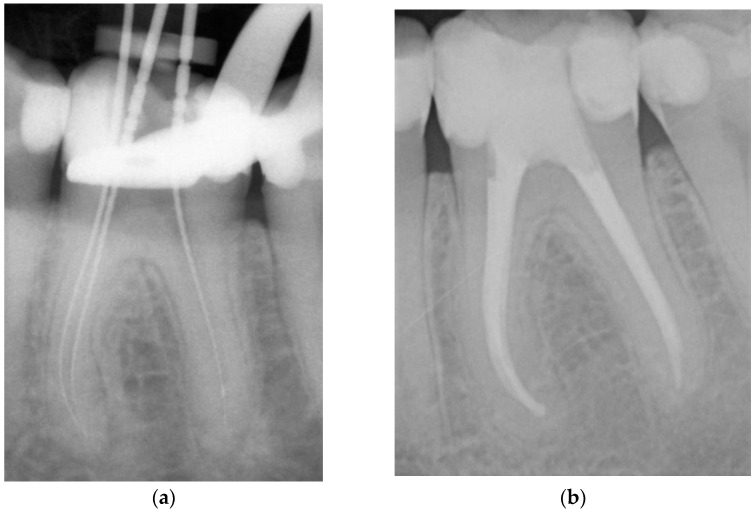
Representative example of root canal filling with Resilon^®^ using the continuous wave of condensation technique (CWR); working length radiograph of tooth 36 (a); 6-year follow-up radiograph showing complete apical healing (b).
